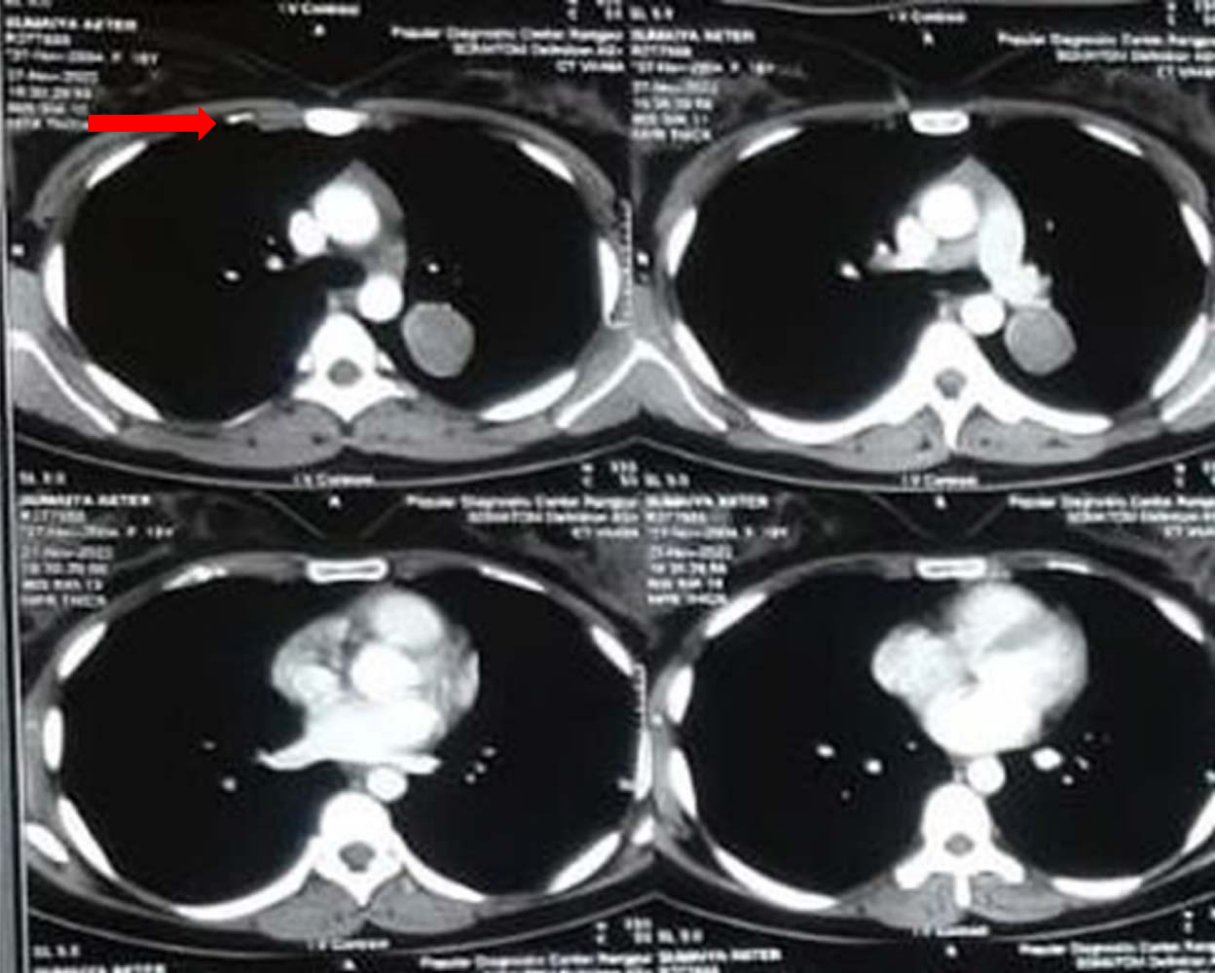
Contrast-enhanced chest CT scan showing a soft-tissue lesion ~3 cm in diameter (red arrow) located in the upper left lobe near the hilar region.
